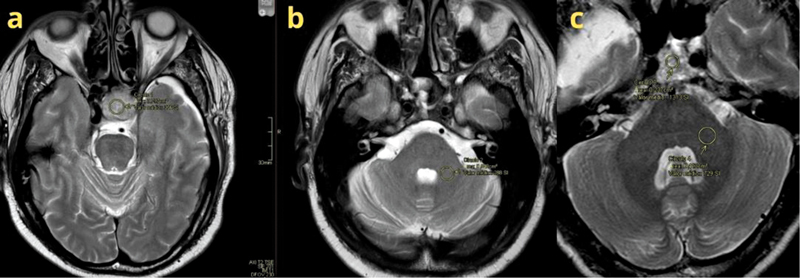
Exemplification of region of interest (ROI) placement for the adenoma-to-cerebellar peduncle ratio (ACP). Magnetic resonance imaging T2-weighted images of patients #1 and #2. 1a) Circle 1–ROI positioned within the homogeneous macroadenoma of patient #1. 1b) Circle 2–ROI positioned in the middle cerebellar peduncle of patient #1. 1c) Circles 3 and 4–ROIs exemplifying placement within the heterogeneous macroadenoma (circle 3) and the middle cerebellar peduncle (circle 4) of patient #2.
